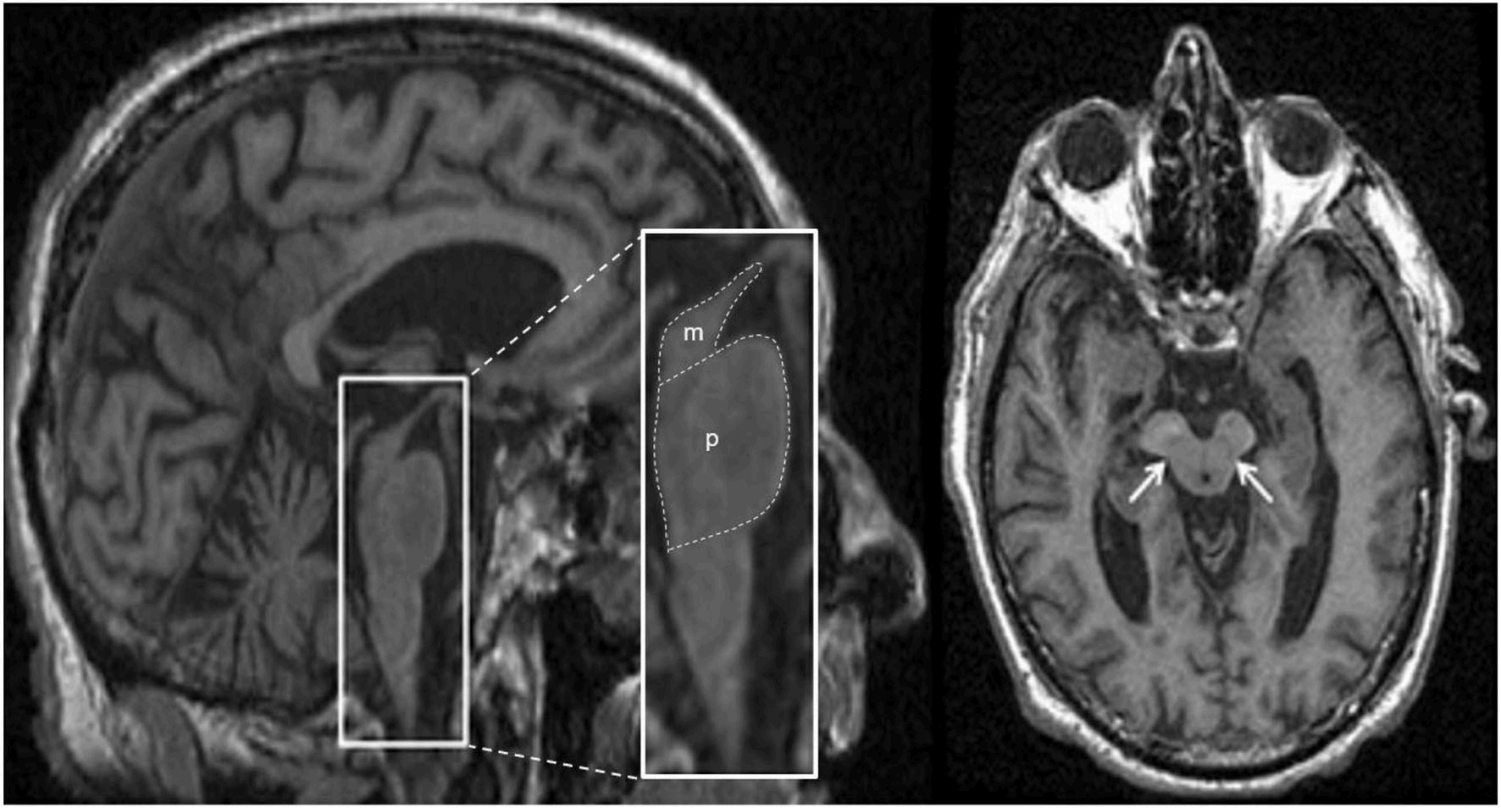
Magnetic resonance imaging of a patient clinically-diagnosed with progressive supranuclear palsy. The left image is a sagittal T1-weighted sequence showing the “hummingbird” sign (smaller box), while the right image is an axial T1-weighted sequence showing the “morning glory” sign (arrows); both features are seen in progressive supranuclear palsy. The pons (p) and midbrain (m) areas are also shown (larger box), and their ratios have been used to calculate an index to assist in the diagnosis (128). Figure adapted from Saeed et al. (10), under the Creative Commons Attribution License 4.0 (https://creativecommons.org/licenses/by/4.0/).
